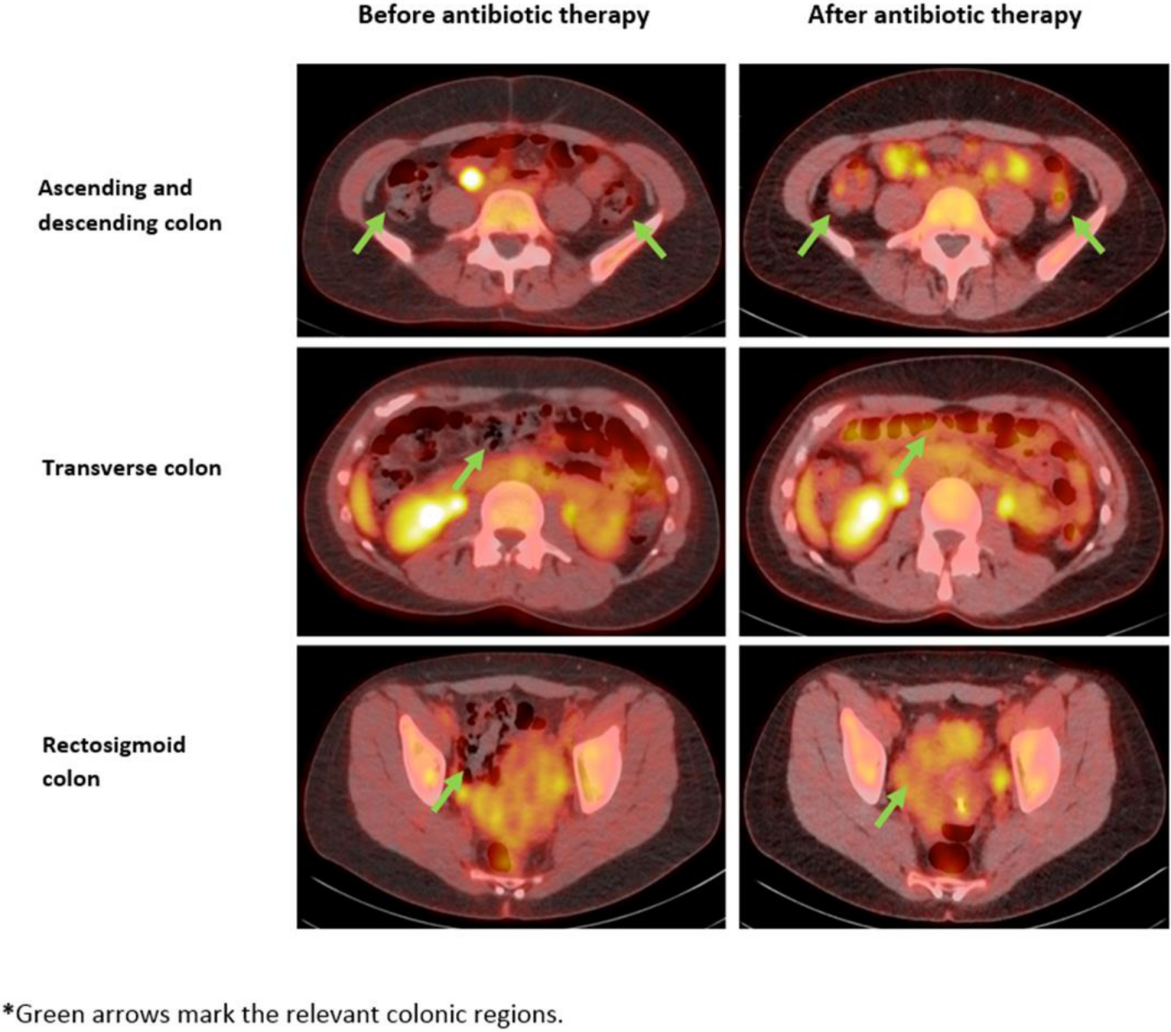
18F-FDG PET uptake in different colonic regions before and after antibiotic therapy in the first individual enrolled to the study.
